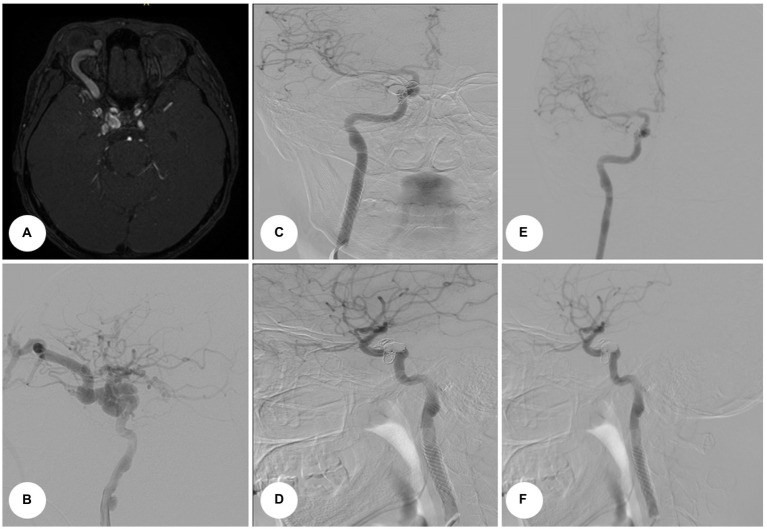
An instrumental examination of Case 2. (A) Axial MRI revealed enlargement of the superior ophthalmic vein and expansion of the right cavernous sinus in a patient with right side carotid-cavernous fistula. (B) Preoperative angiogram. (C) Postoperative control angiograms reveal stent deployment and aneurysm exclusion from the blood circulation. Anterior–posterior projection. (D) Lateral view. (E) Post-procedural angiograms (follow-up after 6 months). Anterior view. (F) Post-procedural angiograms (follow-up after 6 months). Lateral view.
